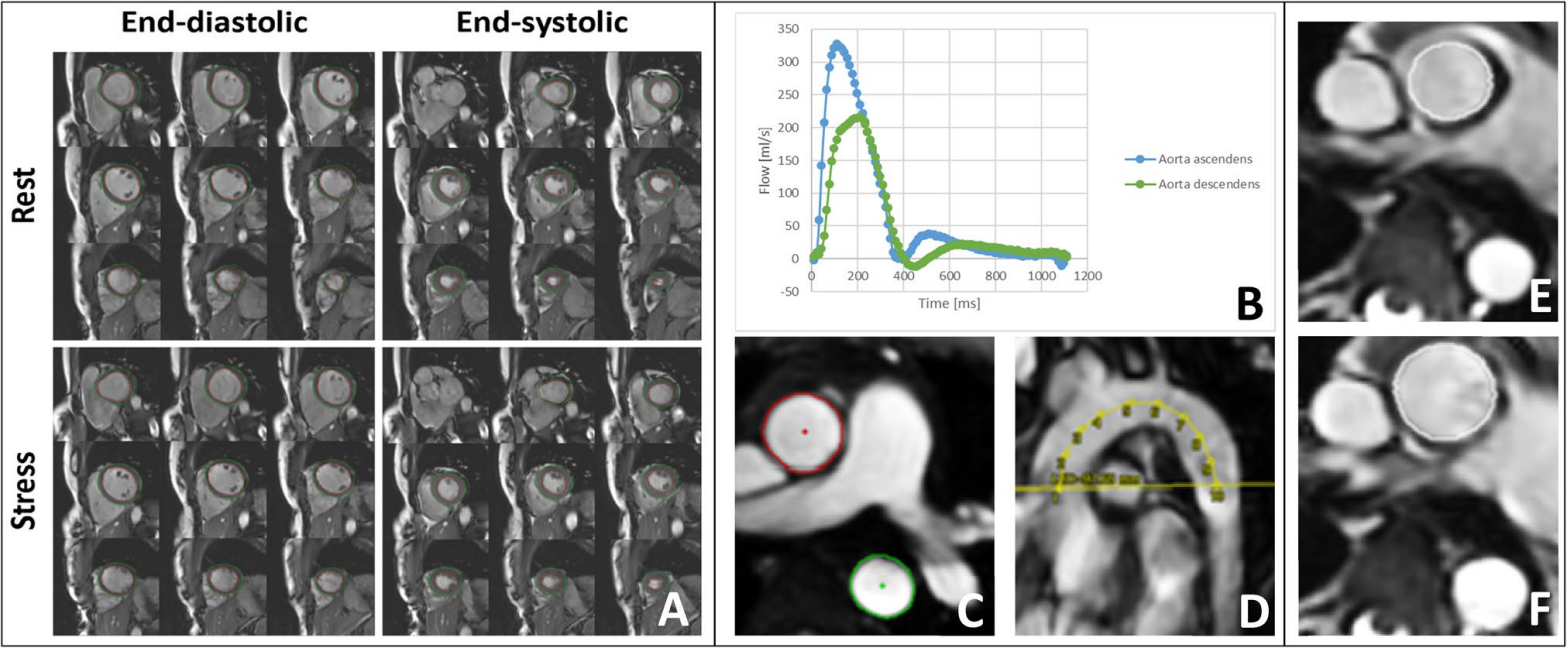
Example of the cardiac MRI images obtained during rest and exercise. A Left ventricle chamber quantification in short axis slices that cover the whole left ventricle. The left ventricle endocardium and left ventricle epicardium are delineated in systole and diastole, during rest and during exercise. B–D. Measurement of the pulse wave velocity (PWV). The contours of the ascending and descending aorta were drawn to measure the blood flow in ml/s (B, C). PWV was calculated as the ratio of distance Δx per time Δt, where Δx is the length of the aortic segment measured on the MR image data along the centerline (D), and Δt is the time duration needed for the pulse wave velocity to travel that length through the aorta. To calculate the Δt, we identified the time point of the maximum flow upslope of the ascending aorta and the maximum flow upslope of the descending aorta and calculated the Δt between these two time points in ms. E–F Calculation of the aortic distensibility. The minimum (E) and maximum (F) ascending aortic cross-sectional areas were measured. Aortic distensibility (10^–3^ mm Hg^−1^) was calculated using the following calculation: (maximum area – minimum area)/(minimum area × ΔP) × 1000, where ΔP is the brachial pulse pressure in mmHg
